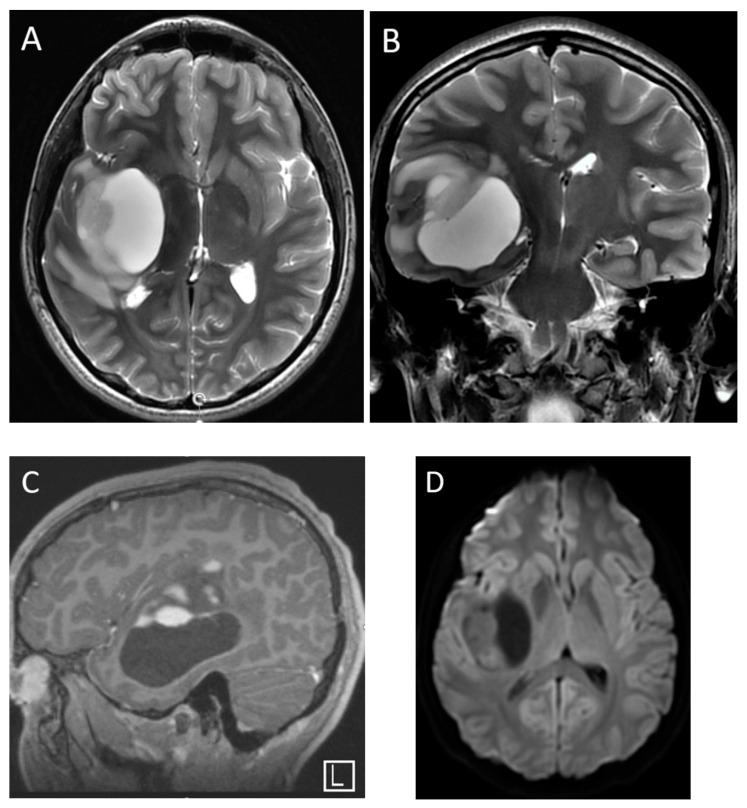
A 16-year-old male with ganglioglioma, presenting with seizures. Axial and coronal T2-weighted (A,B), sagittal post-contrast T1-weighted (C) and axial DWI (D) images demonstrate a large predominantly cystic mass with eccentric solid component in the right temporal lobe. There is patchy but avid enhancement within the solid component. Lack of diffusion restriction within the solid component helps distinguish this from higher grade tumors.
